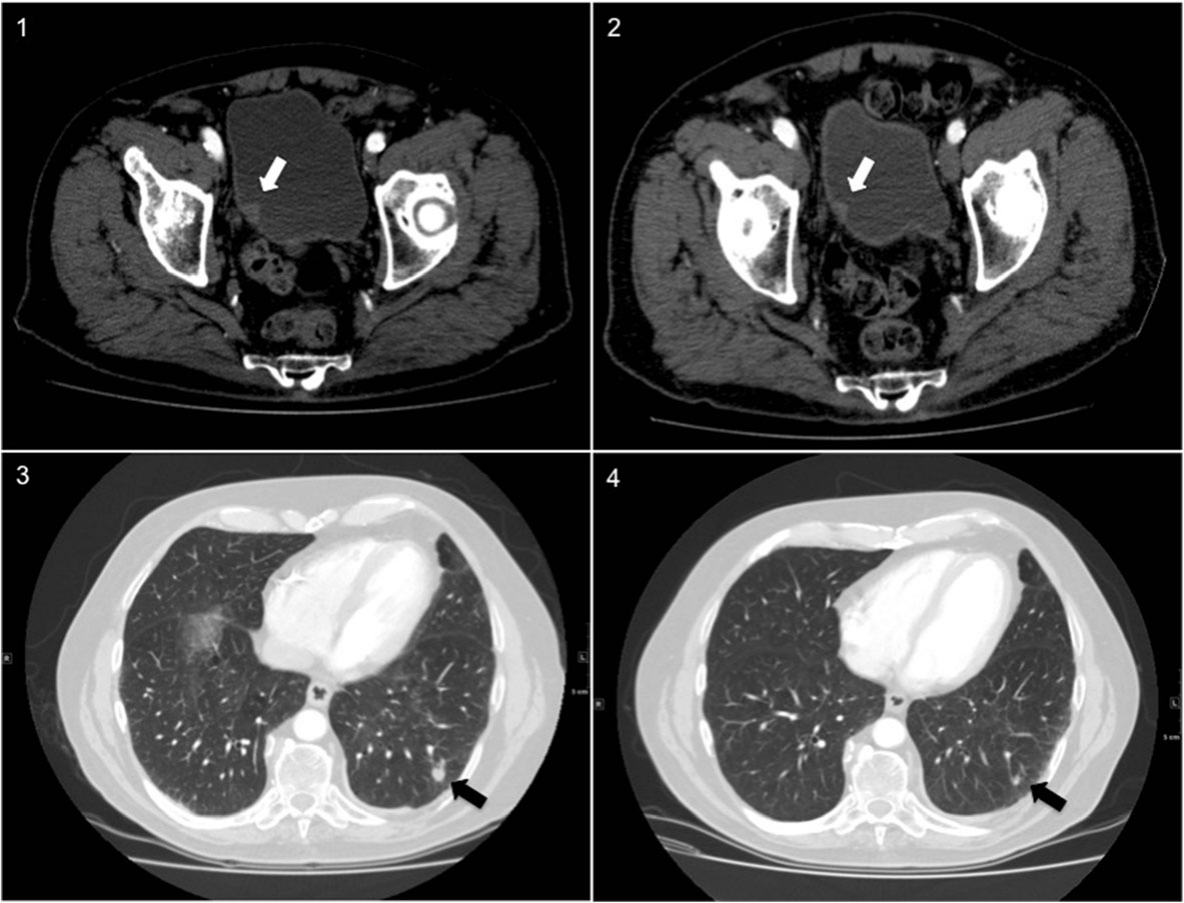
Example images of missed malignant findings on surveillance CT Angiogram post endovascular aortic aneurysm repair. Axial slice of CTA showing the urinary bladder right lateral wall thickening (white arrow), confirmed bladder transitional cell carcinoma at diagnosis (1) and retrospectively on a previous scan (2). Axial slice of CTA showing the 11 mm left lung lower lobe lesion (black arrow), confirmed adeno-squamous lung cancer at diagnosis (3) and retrospectively on a previous scan (4), which was initially considered benign
